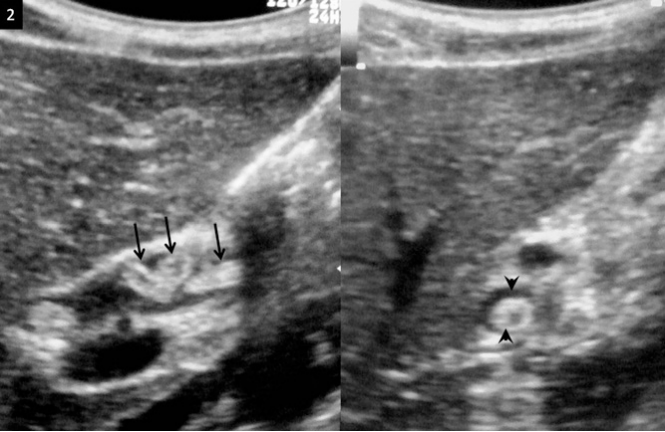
Ultrasound image of common bile duct showing *Ascaris* as tubular structure within the lumen and target sign on cross-section.
